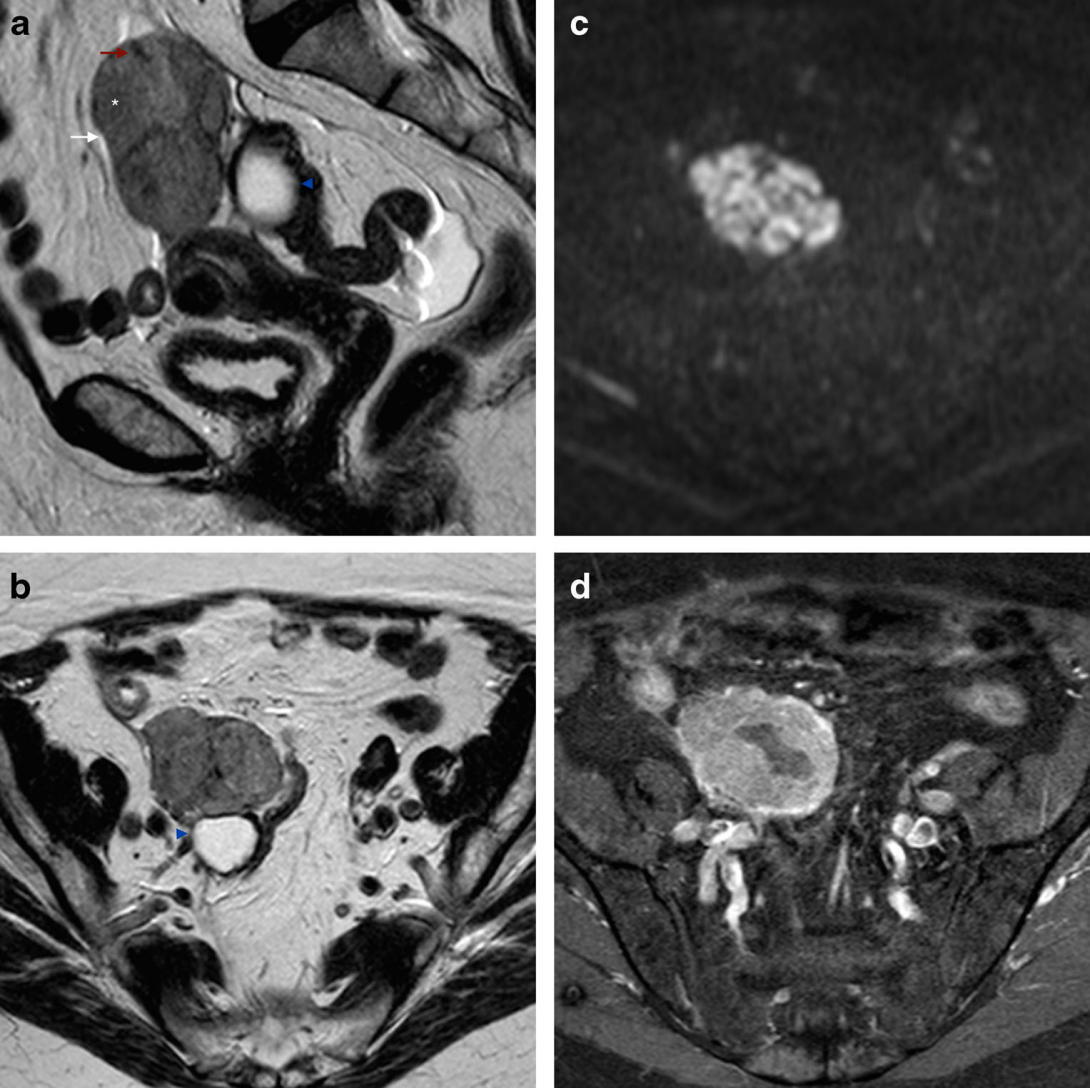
Serous PFTC in a 78-year-old female, presenting with postmenopausal bleeding and pelvic pain. a Sagittal T2WI showing a solid mass (*asterisk*) with well-defined walls (*white arrow*), incomplete folds (*red arrow*), and an incidental cystic lesion corresponding to a cystadenofibroma (*blue arrowhead*); b Axial T2WI showing the ovarian cystadenofibroma, posterior to the mass (*blue arrowhead*); c Axial DWI with the same solid mass showing restriction to diffusion; d Axial T1WI, fat-saturated, contrast-enhanced image, showing contrast uptake by the mass, with central necrosis
